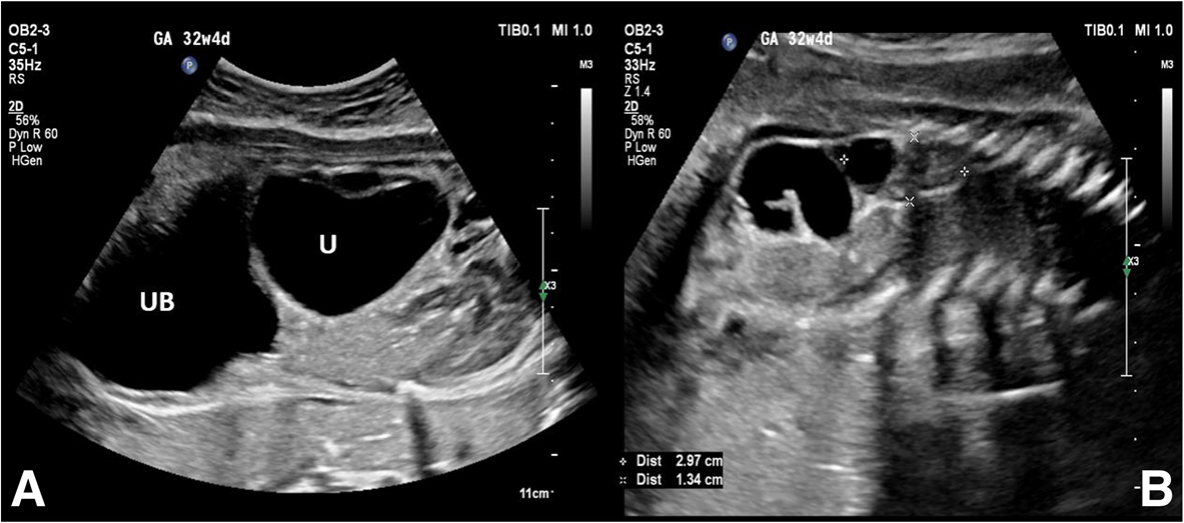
Two pictures of ultrasound at 32 weeks and 4 days of gestation in which: a an axial view of two-dimensional ultrasound shows (*UB*) progressive and severe enlargement of urinary bladder, and (*U*) progressive enlargement of ureter; b a coronal view of two-dimensional ultrasound shows a hugely distended abdomen and anhydramnios. *U* ureter, *UB* urinary bladder
